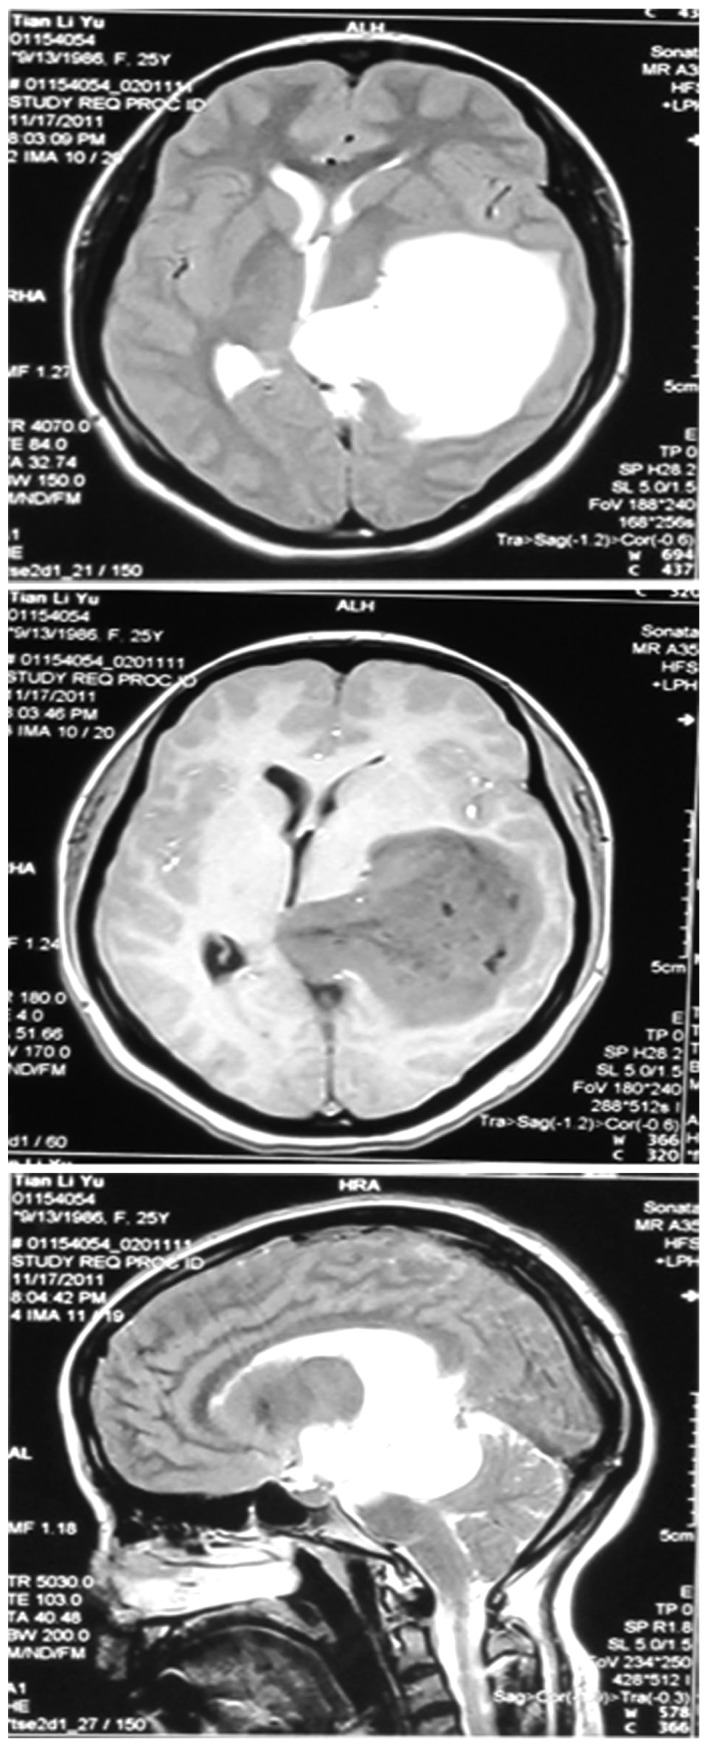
Images from a magnetic resonance imaging (MRI) scan of case 1.
